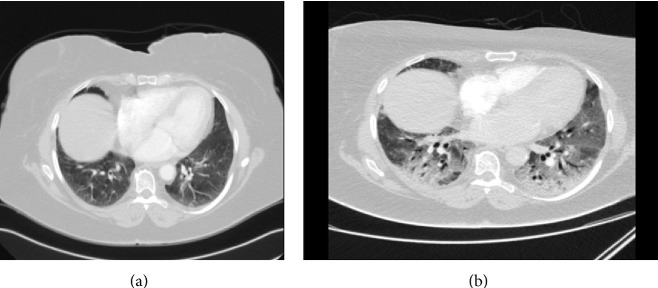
(a) Chest CT scan of patient 1, three months prior to symptoms of pneumonitis. (b) Chest CT scan of patient 1, at the beginning of respiratory symptoms: diffuse interstitial infiltrates and ground glass opacities shown in both lung fields.
